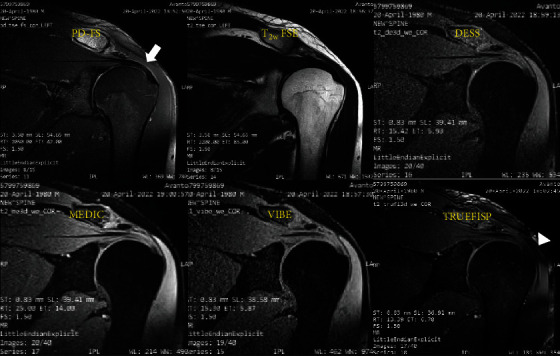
Conventional 2D and 3D MRI images of the right shoulder joint in a 44-year-old female. PD-FS image shows magic angle artifact (arrows). The TRUEFISP image reveals susceptibility artifact (arrowhead), whereas other 3D sequences show no artifacts.
